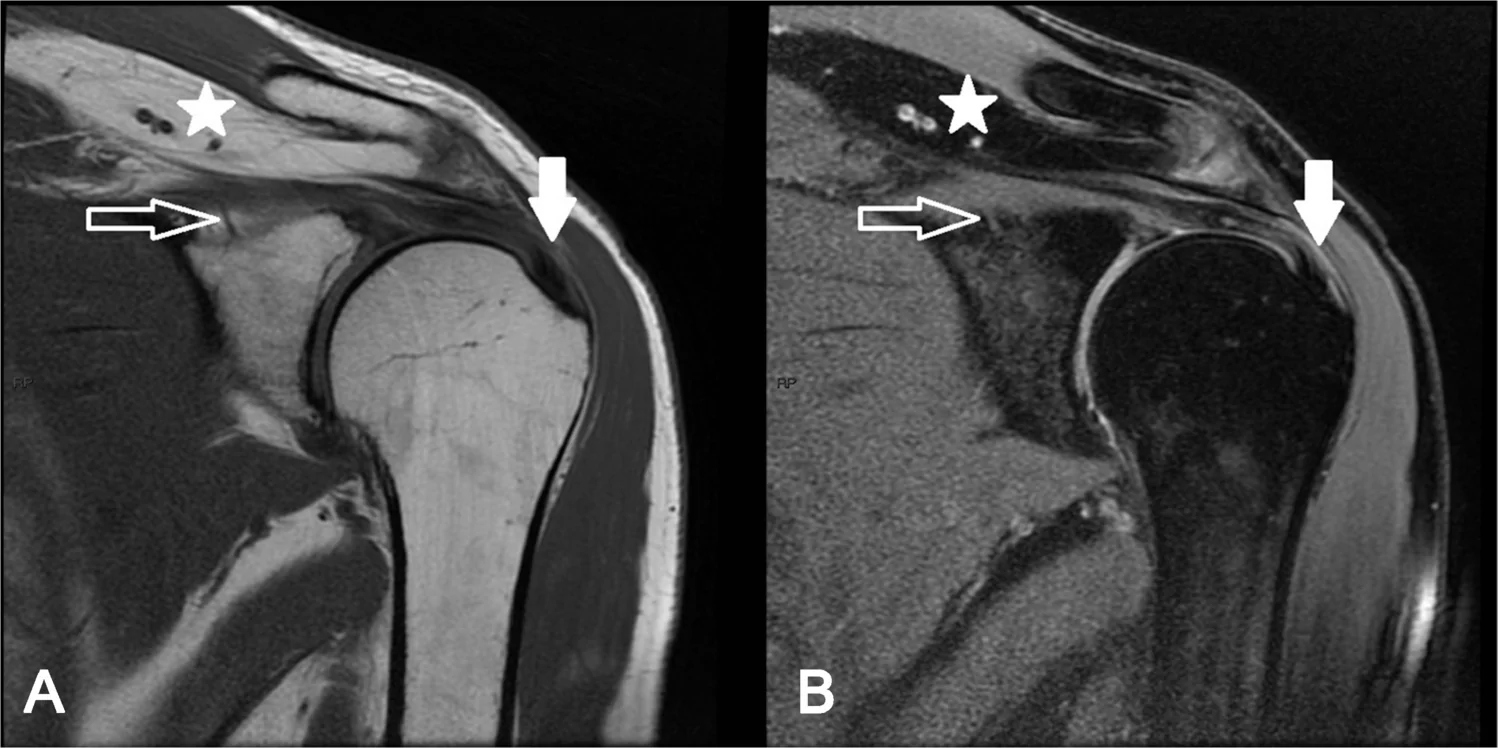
MR of the shoulder at the Suprascapular notch. Coronal oblique T1-weighted image (A) and corresponding Proton Density with Fat Saturation (B) demonstrates normal morphology and signal of the suprascapular nerve at the suprascapular notch (white open arrow). Adjacent severe fatty atrophy of the supraspinatus muscle (white solid star) is present and the intact distal supraspinatus tendon insertion shows mild tendinosis (white solid arrow)
